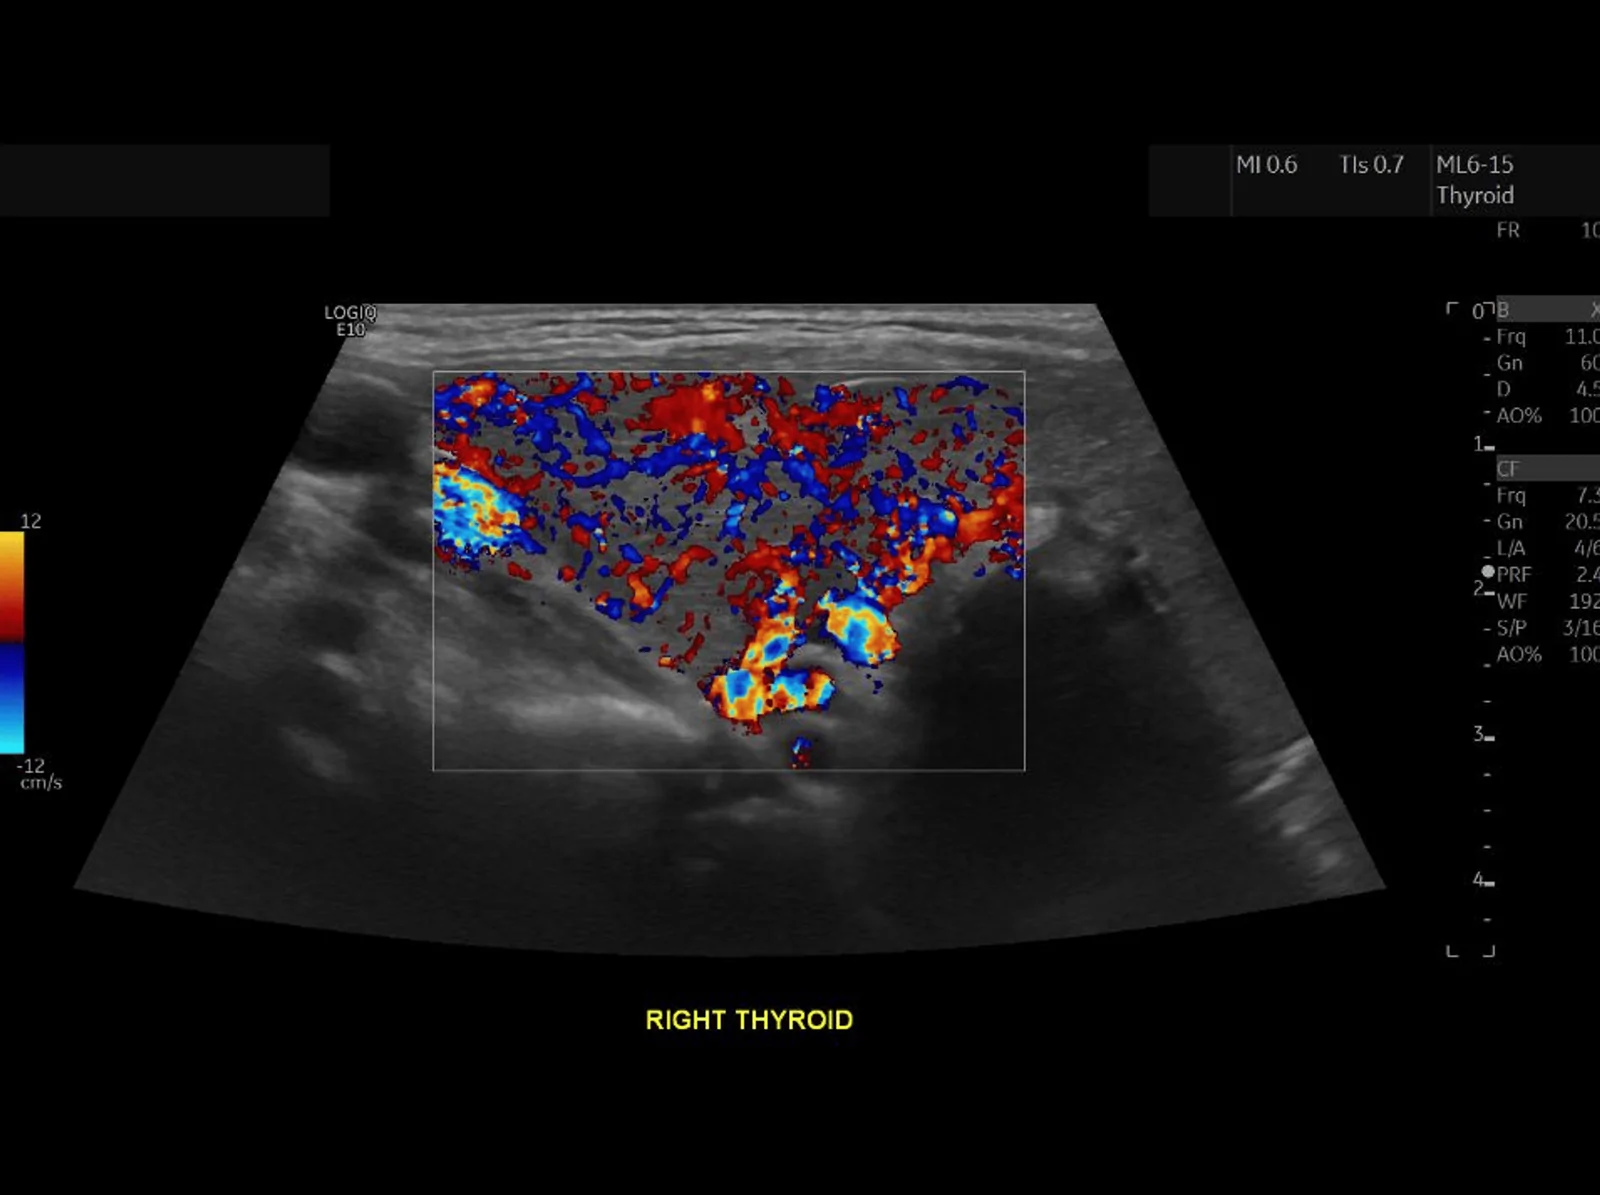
Color Doppler ultrasound of the right thyroid lobe demonstrating markedly increased vascularity, consistent with active thyroiditis.
